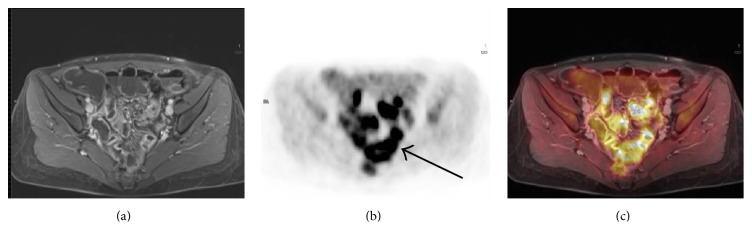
Simultaneous ^18^F-FDG PET/MR in a patient with known Crohn's disease. (a) Axial T1-weighted fat-saturated postcontrast images with mucosal hyperenhancement of several loops of small bowel in the lower abdomen consistent with active inflammation. (b) Attenuation-corrected PET images demonstrate intense ^18^F-FDG uptake in these bowel loops. (c) Fusion overlay images demonstrate localization of ^18^F-FDG avidity to the enhancing small bowel loops. Images courtesy of Onofrio Catalano, MD.
